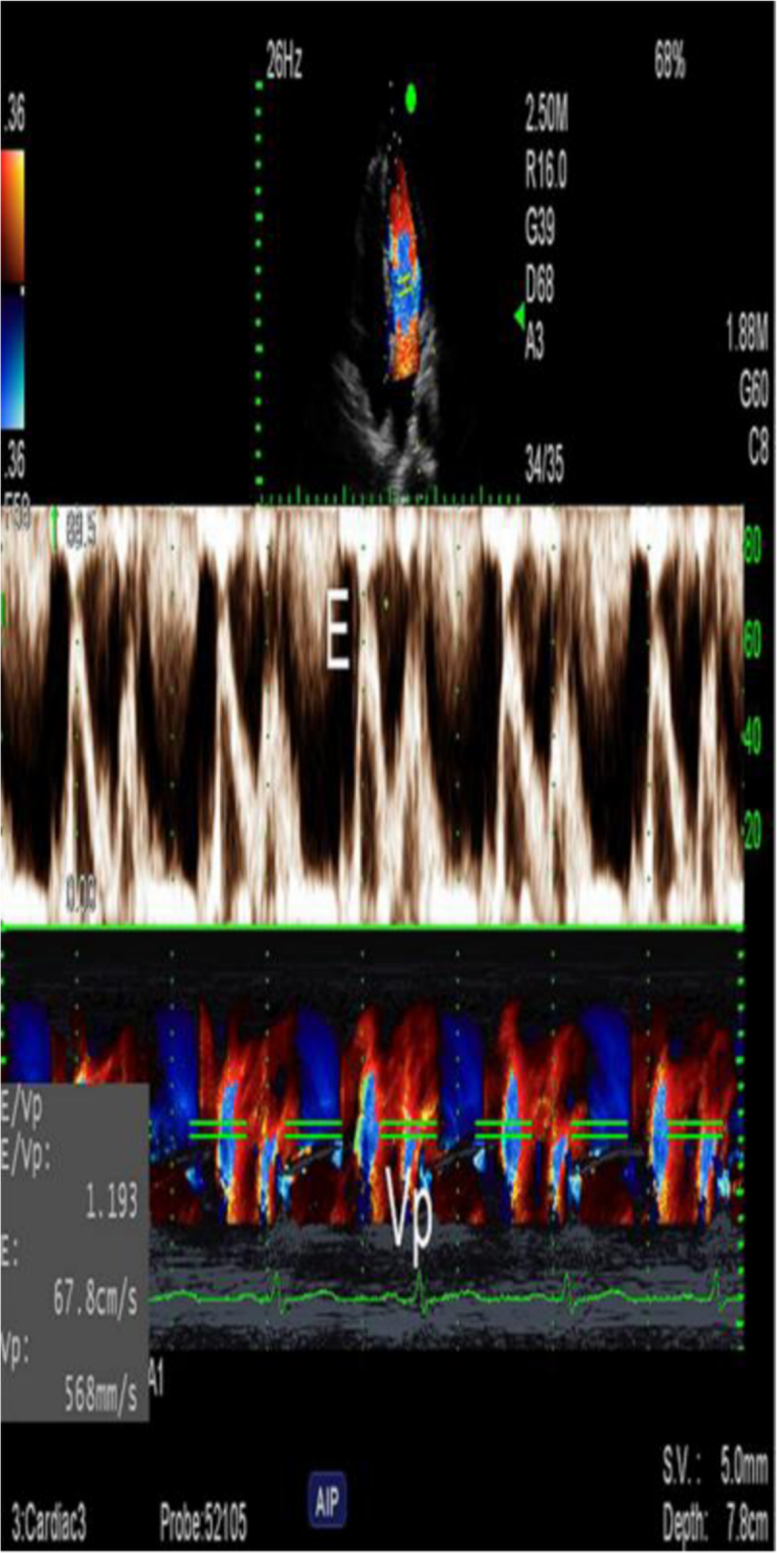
Early transmitral flow peak velocity(E) and Vp were measured in the same cardiac cycle using M-PW mode echocardiography. Vp was measured as the slope of the first aliasing velocity during early filling, from the mitral valve plane to 4 cm distally into the left ventricular cavity. E/Vp is the ratio of E to Vp
